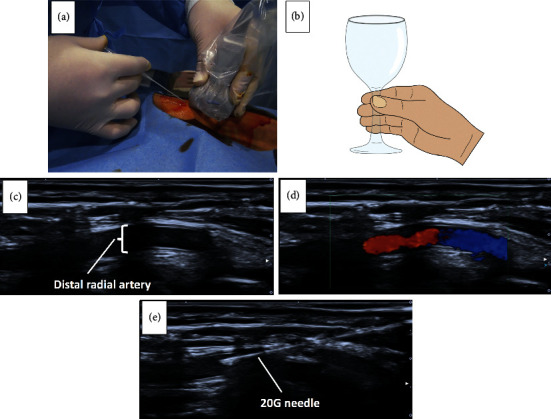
(a) A puncture with a 20 G needle under the long-axis ultrasound guidance. (b) The way of using the patient's hand that has a wineglass makes us puncture under ultrasound guidance easily. (c) Long-axis ultrasound shows the distal radial artery. (d) The Doppler-ultrasound shows the blood flow in the radial artery. (e) Ultrasound shows the puncture with a 20 G needle.
